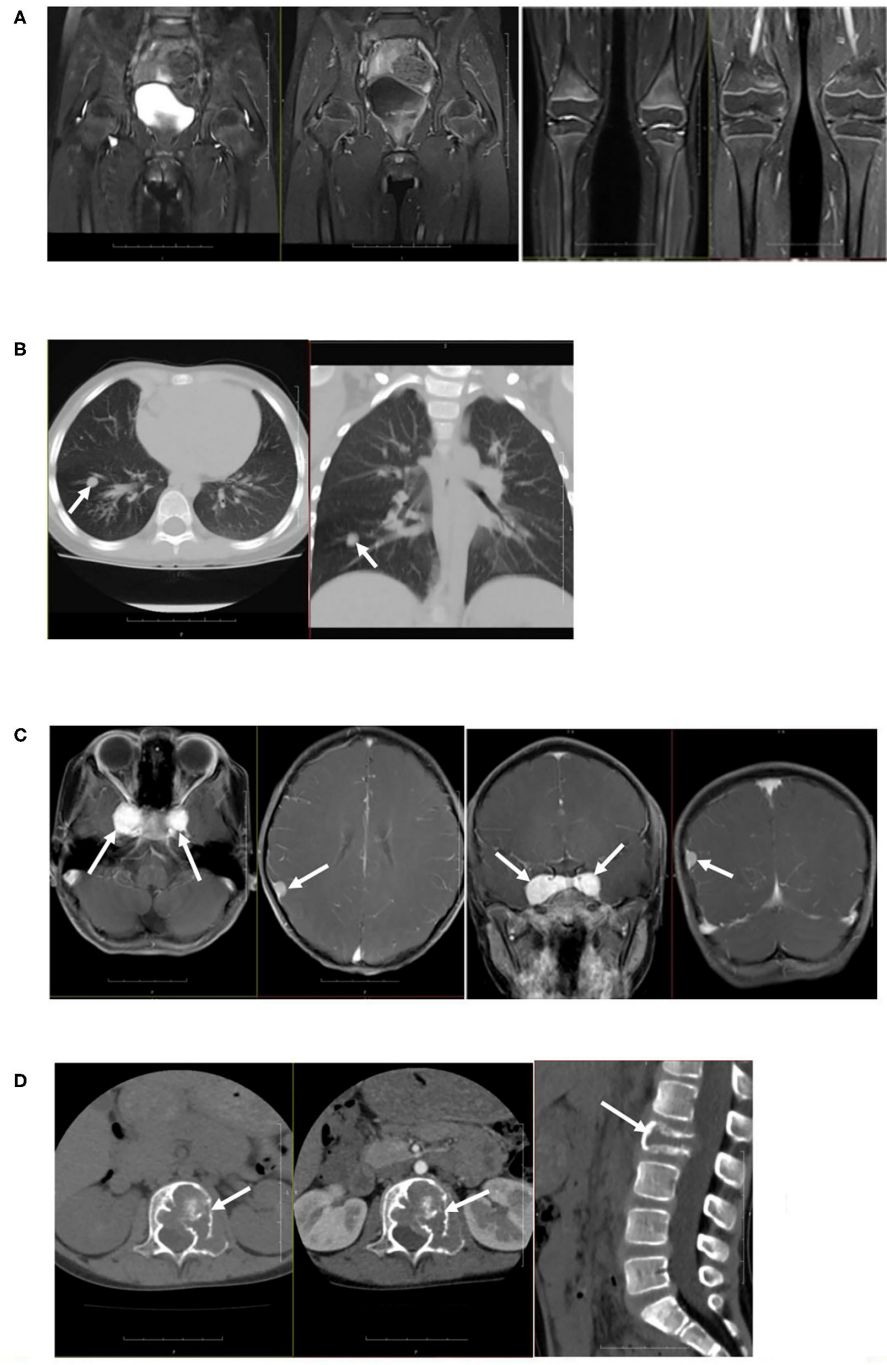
Imaging examination. (A) Plain and enhanced MRI scanning of the knee joints and hip joints showed that the synovium of bilateral knee and hip joints were slightly thickened and enhanced, with a small amount of effusion in joints. (B) Plain CT scanning of lungs showed multiple nodules and stripe-shaped shadows in bilateral lungs, and the size of the largest shadow was 0.63 × 0.74 cm. (C) Plain and enhanced MRI scanning of the skull showed nodular lesions at the parasellar and meninges of the right parietal lobe. (D) Plain and enhanced MRI scanning of the spine showed bone mass destruction of the L2 vertebral body and left vertebral pedicle.
